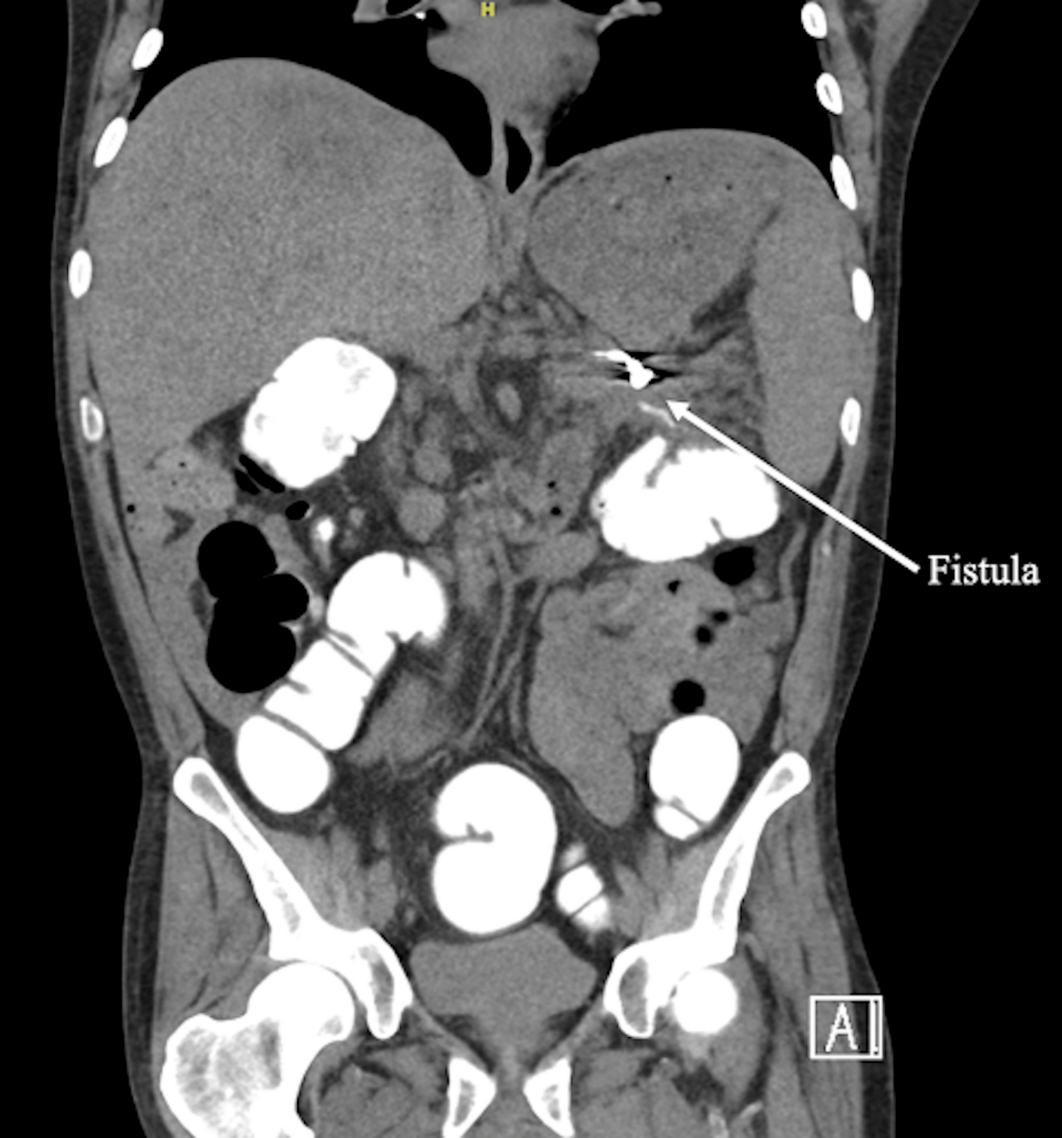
Sagittal CT scan with rectal contrast demonstrating extravasation of contrast (arrow) from the splenic flexure of the colon into the retroperitoneal inflammatory collection, confirming a pancreatico-colonic fistula
